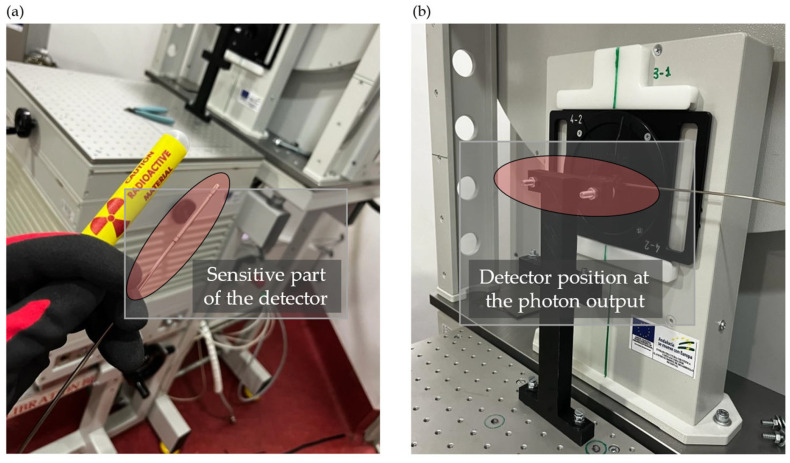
Laboratory of the CIBM for X-ray irradiation: (a) sensitive part of the detector in the foreground. X-ray tube with the support structure anchored to the bench in the background. (b) Detector placed in the irradiation position at the output of the source.
